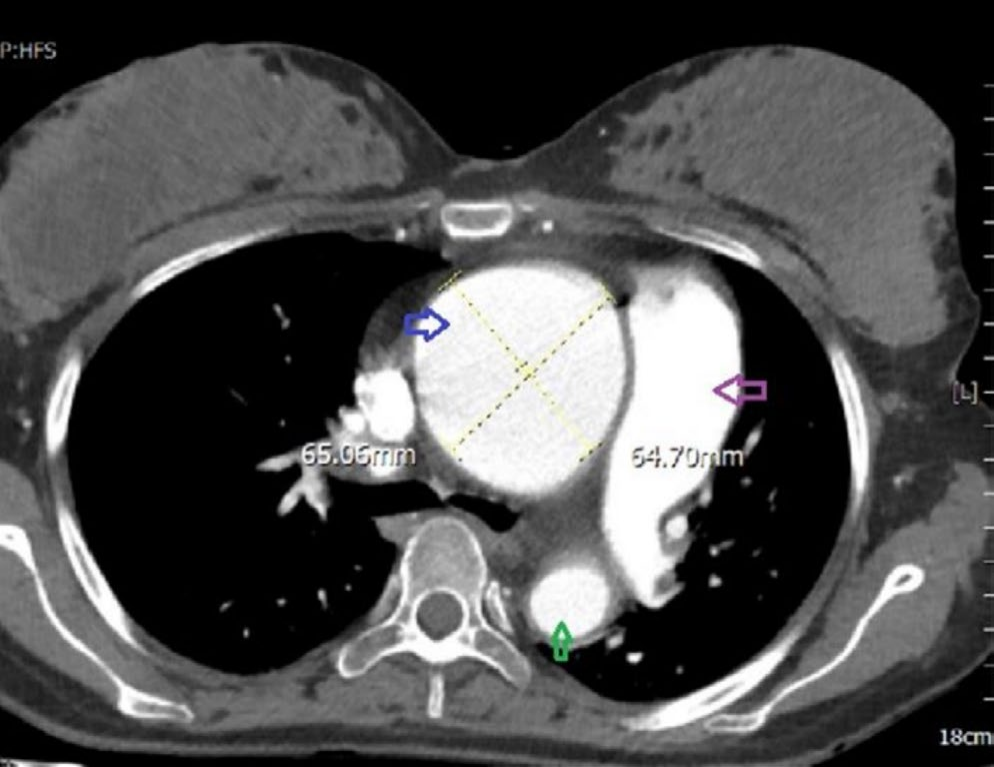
Axial view of thoracic aorta CT angiography after pregnancy. Blue arrow: aneurysm of ascending aorta; green arrow: descending thoracic aorta; purple arrow: main pulmonary artery trunk.
